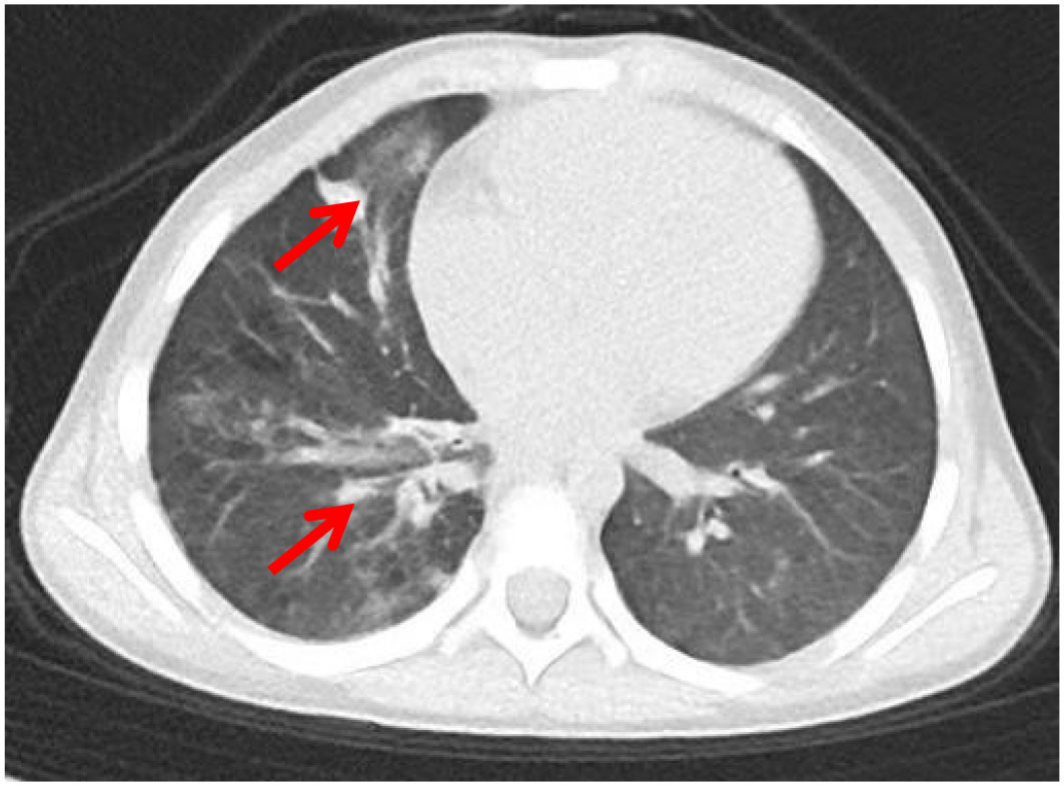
Post-transplant chest CT of the patient (red arrows indicate lesion resolution).
